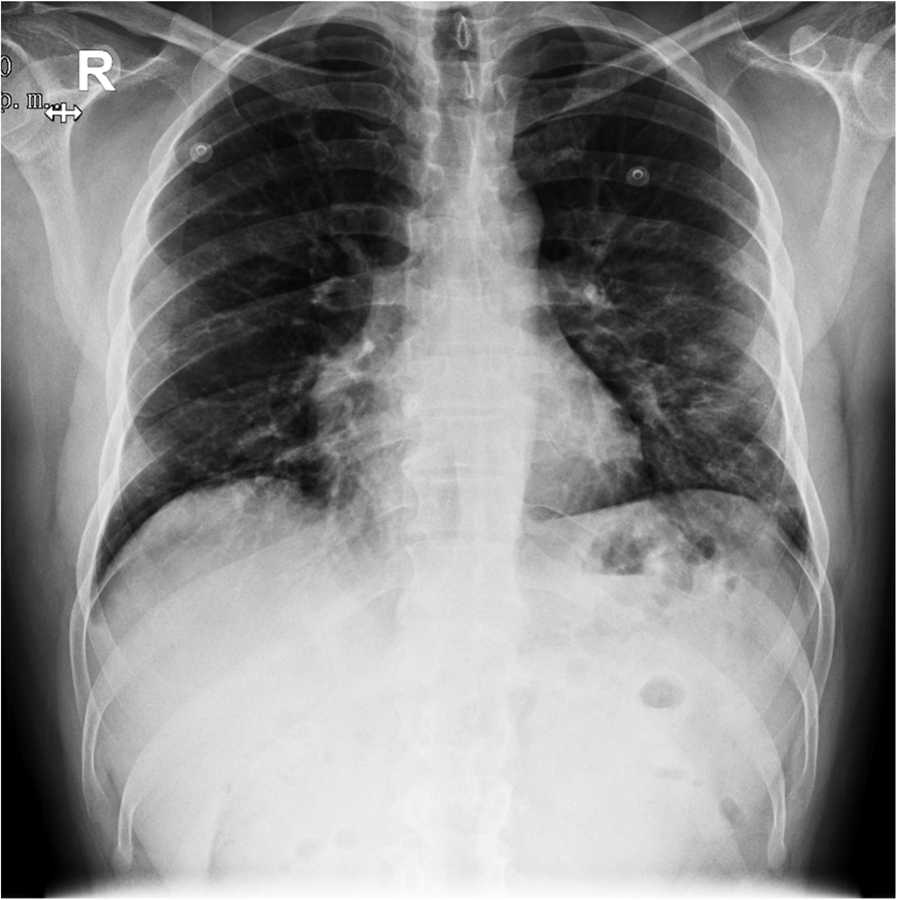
Chest X-ray showing multiple ground-glass bilateral infiltrates
